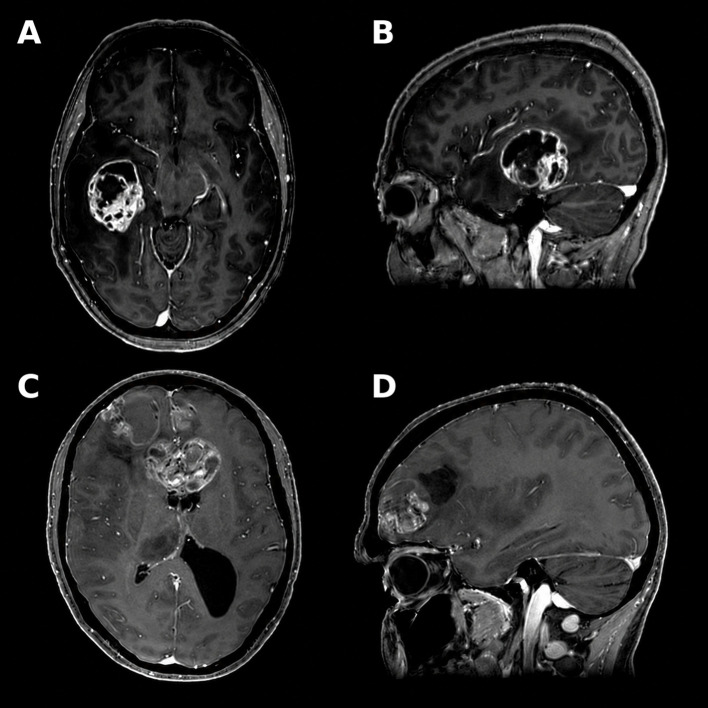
Preoperative brain MRI.*
